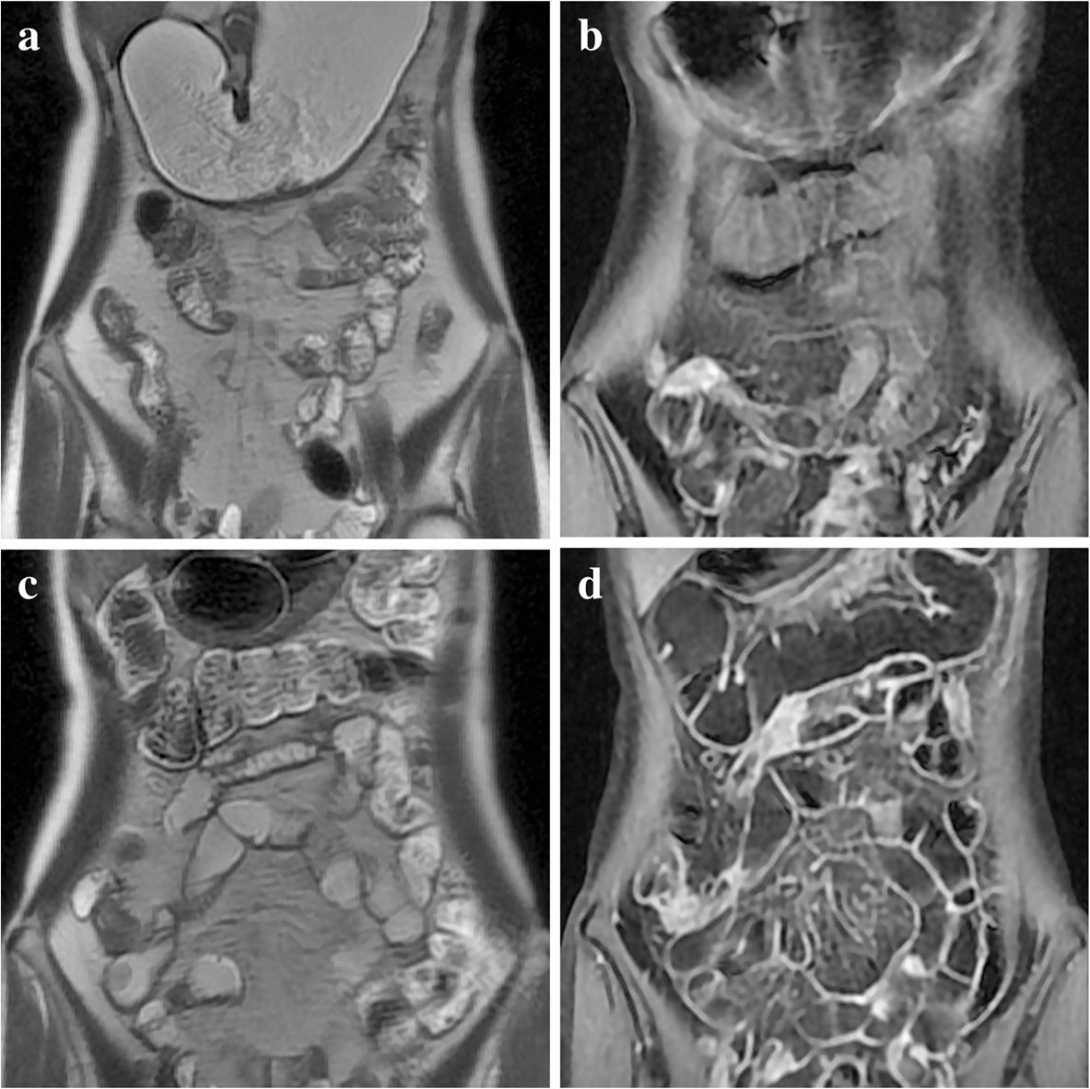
MR images. A 30-year-old woman with CD for eight years has a big polyp located at the duodenum inducing luminal stricture. Coronal T2-weighted SSFSE MRE (a, c) and coronal T1-weighted enhanced MR (b, d) are shown above. a and b are prepared through oral administering: retention of large volume contrast solution in the stomach and collapsed bowel segments representing terrible bowel distention. c and d are prepared through mid-gut tubing: good distention of small bowel
